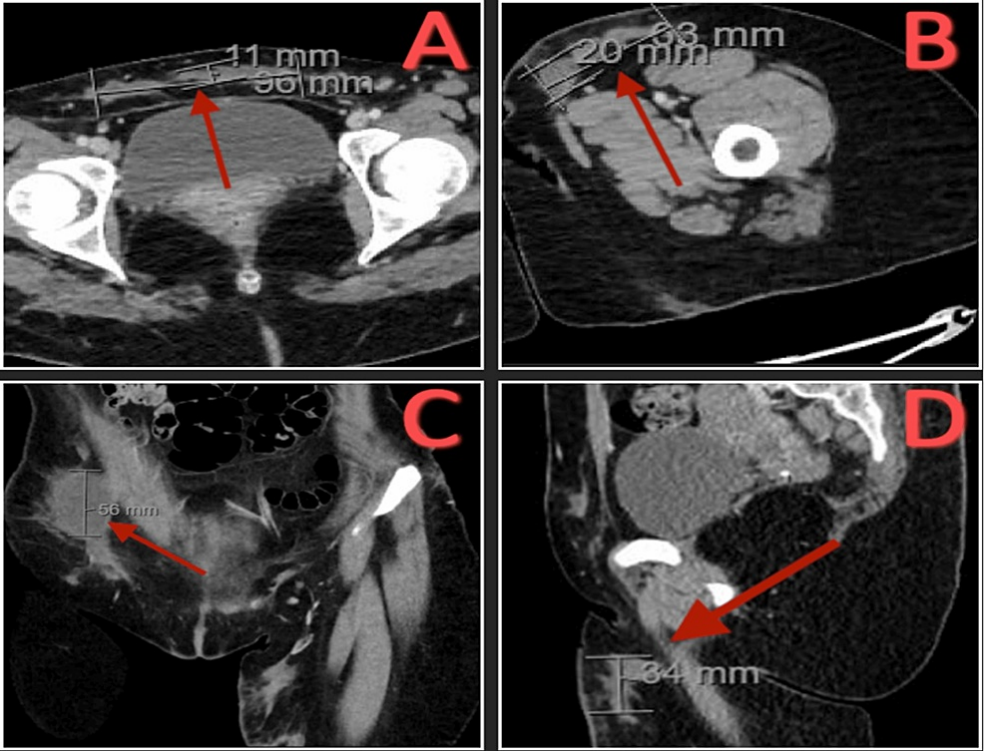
CT pelvis with contrast. (A) Transverse view of an 11 x 96 mm abscess in subcutaneous fat of the lower anterior abdominal wall. (B) Transverse view of a 20 x 63 mm abscess in the subcutaneous fat of the left proximal thigh. (C) Coronal view of 56 mm abscess in the subcutaneous fat of the right hip. (D) Sagittal view of a 34 mm abscess in the subcutaneous fat of the gluteal region.
